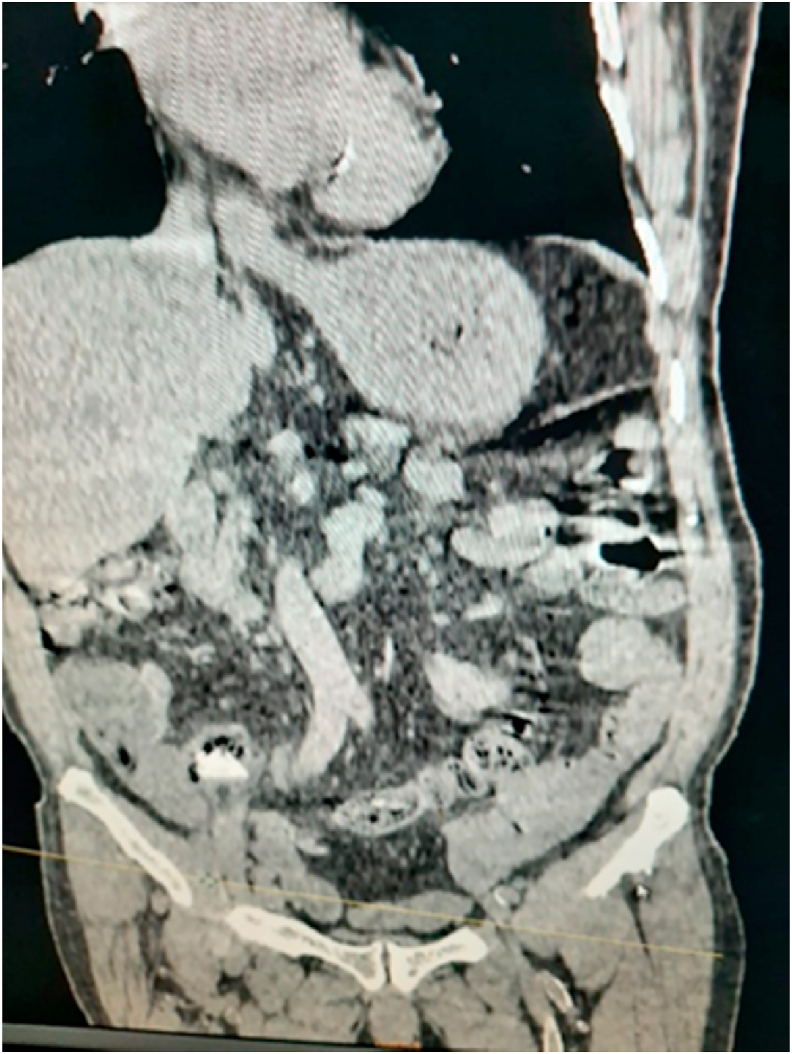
Coronal contrast-enhanced CT-scan of the abdomen showing a hyperdensity indicative of an ingested fish bone.
